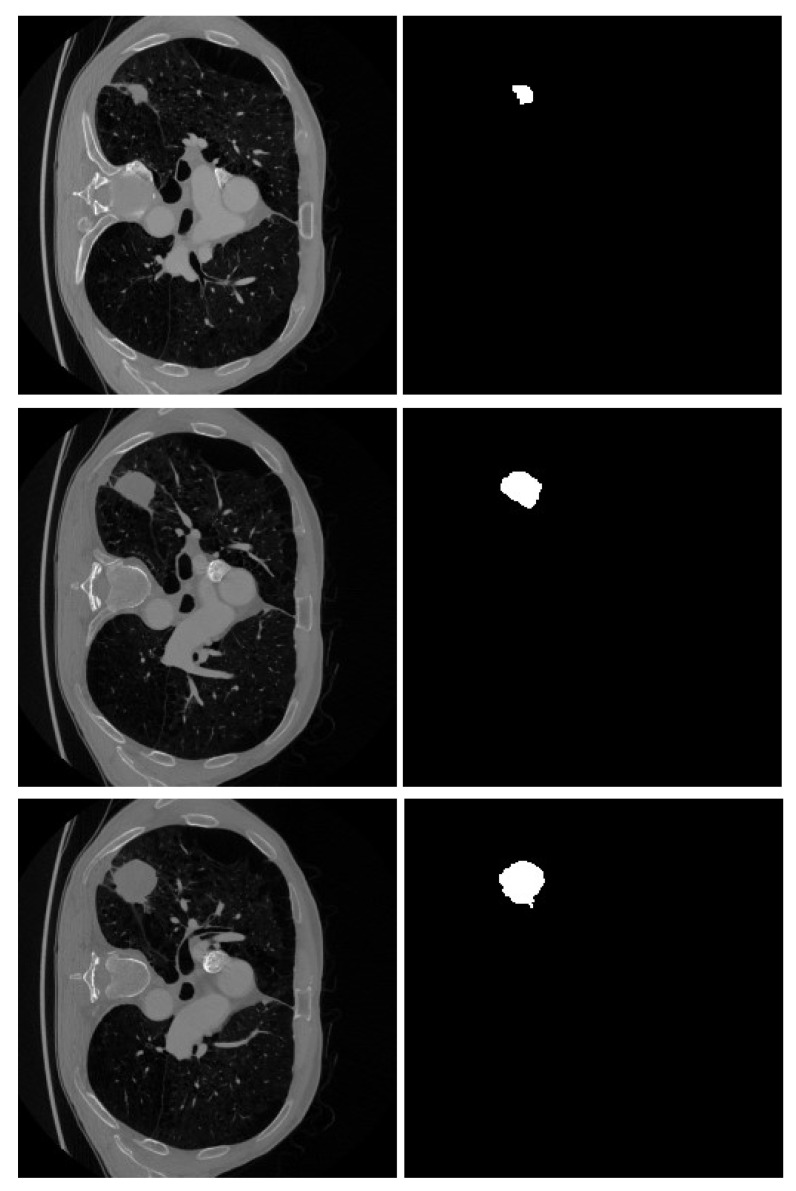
Some samples of lung CT images and their region of tumor as a black-white mask.
